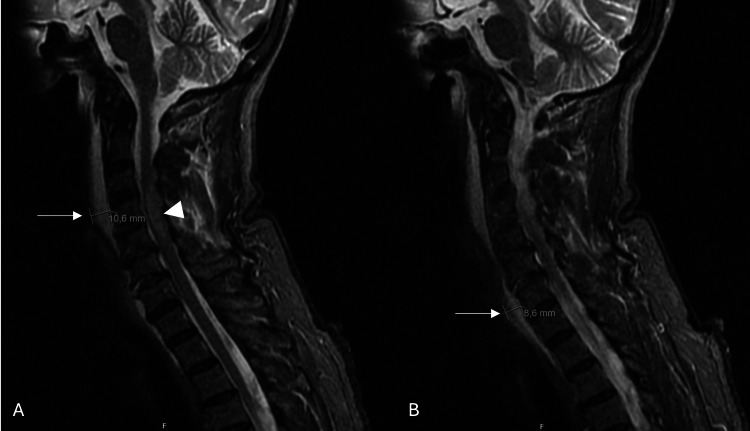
Sagittal T2-weighted MR images of the cervical spine. (A) and (B) demonstrate a prevertebral hematoma anterior to the mid-cervical vertebral bodies, with maximal thickness measurements of 10.6 mm and 8.6 mm in different sagittal planes (arrows), and associated spinal cord edema/contusion (arrow tip) at the C4–C5 level.
